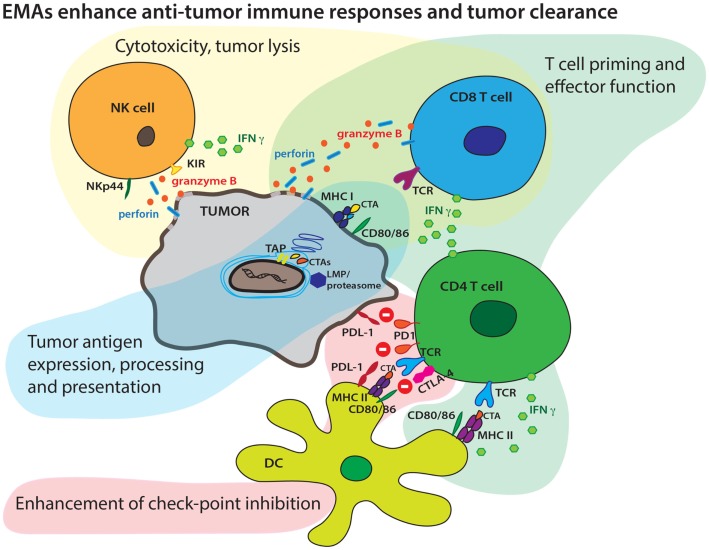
Epigenetic modifying agents can enhance multiple aspects of an antitumor immune response. EMAs may boost tumor antigen expression, endogenous antigen processing, increase surface CTA display in context of MHC molecules, and boost presentation to T cells by increasing expression of co-stimulatory molecules. EMAs may also enhance both cellular and cytokine-mediated effector T-cell mechanisms and tumor lysis. EMAs may alter checkpoint inhibition targeting the PD1/PD-L1 and CTLA-4/CD28 axis resulting in more efficient effector T-cell mechanisms.
